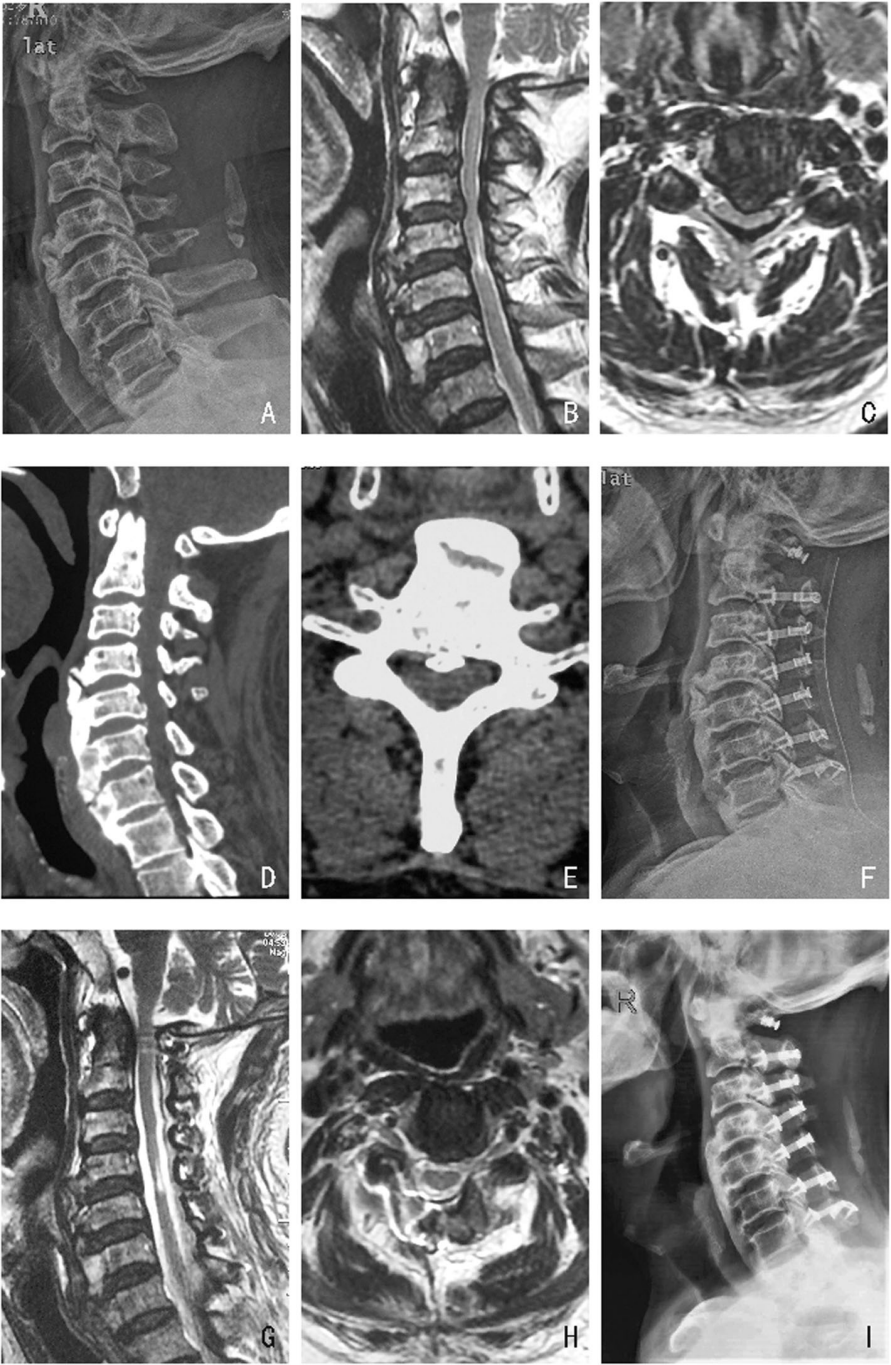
a, f, i A comparison of the changes in cervical alignment among measurements obtained preoperative, postoperative and 1 year after surgery in radiographs obtained in a 62-year-old male patient who underwent EODL extended to C1. b-e Preoperative MRI and CT. g-h Postoperative MRI
